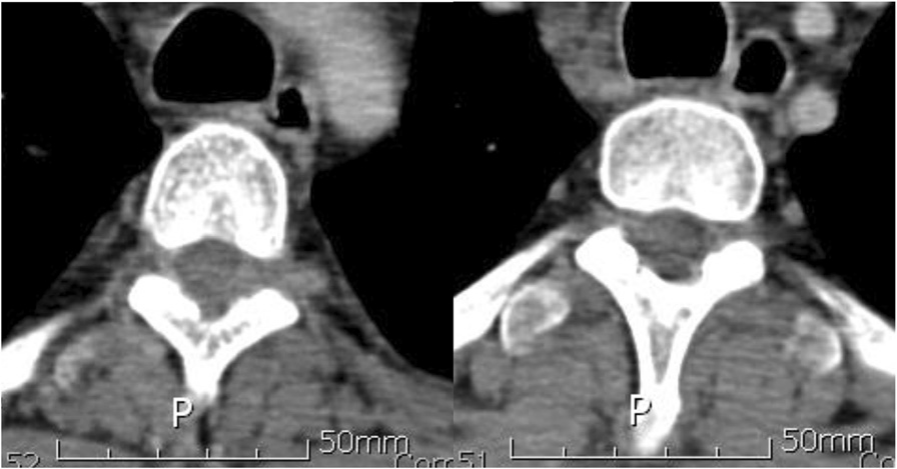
Computed tomography shows no bony destruction at the T2 to T4 levels.
